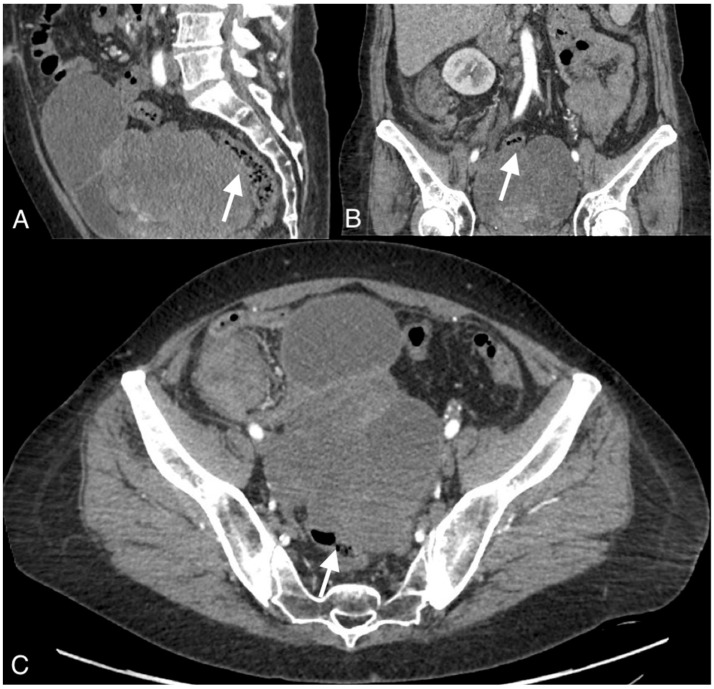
Images of a 54-year-old patient with an ovarian cancer recurrence in the form of the fistula between the tumor and rectum. CT obtained in the coronal (A), sagittal (B), and axial plane (C) shows large pelvic tumor with adjacent rectum.
